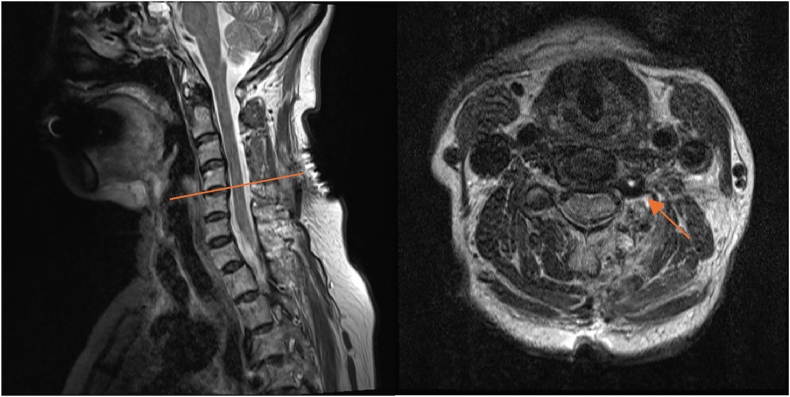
Postoperative MRI sagittal and axial cut showing adequate decompression of the nerve.
